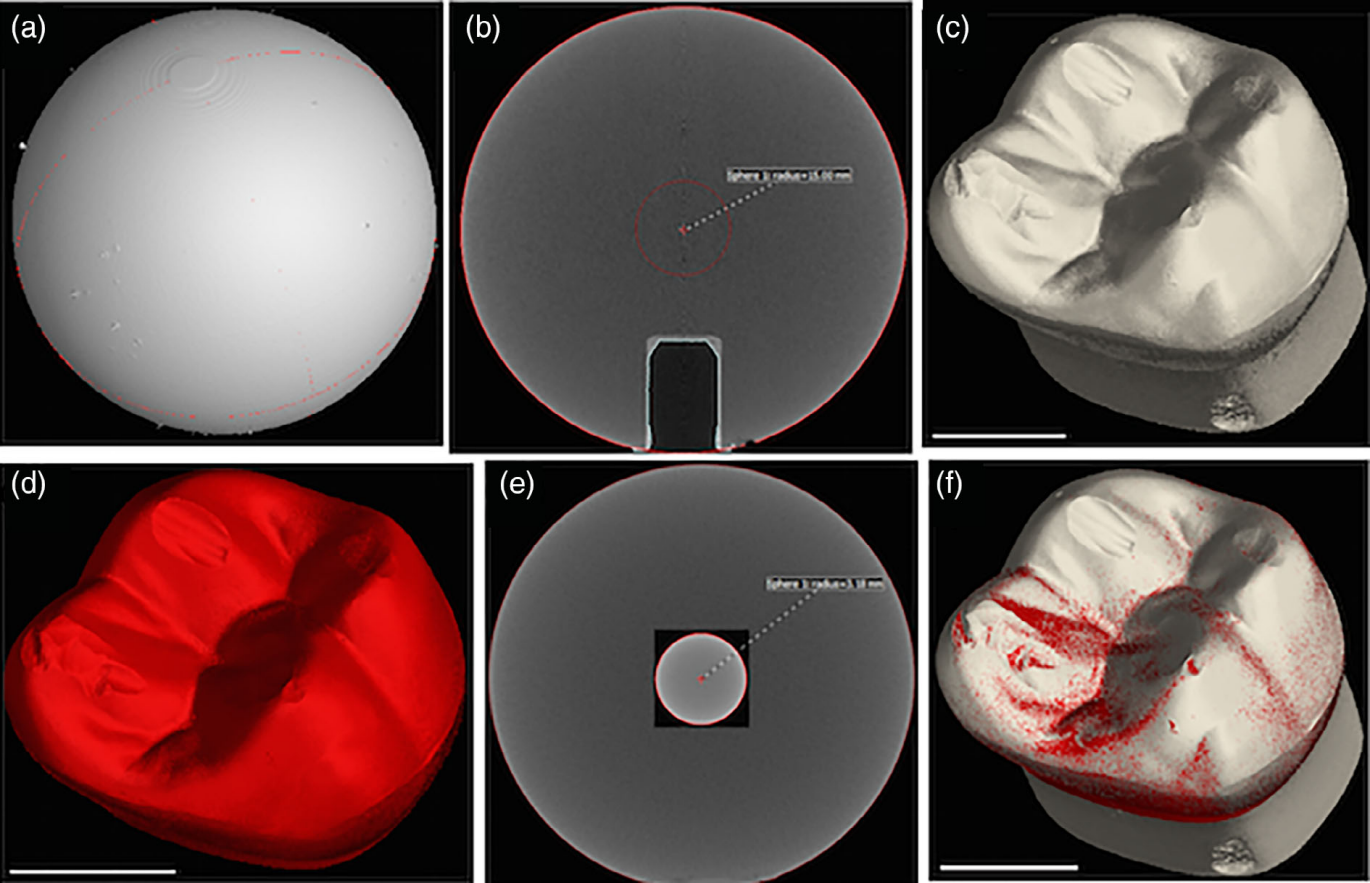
(a) 3D volume reconstruction of a 30‐mm ceramic sphere scanned using the same CT scan position selected for scanning the Dentoform® samples; (b) Front view of a tomography image showing as a sphere radius *r* = 15 mm; (c) Representative 3D visualization for the initial worn Dentoform® tooth scanned; (d) Representative 3D visualization for the worn Dentoform® tooth scanned for validation purposes; (e) Front view of a tomography image showing a silicon‐nitride ceramic ball having roughly comparable size and density as the Dentoform® samples; (f) Merging two surfaces obtained for initial scanned worn Dentoform® and rescanned worn Dentoform®. All reconstruction and segmentation analysis were made using VGStudio Max 3.0. Scale bar 5 mm (white line)
